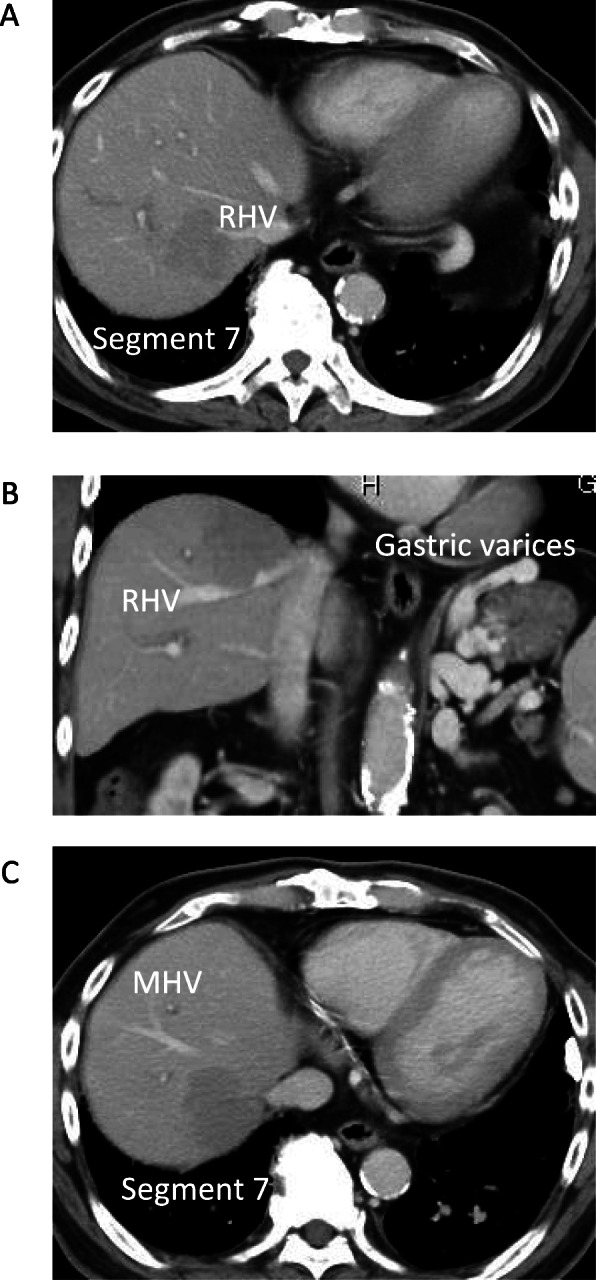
CT imaging of HCC. CT showing the tumor located in segment 7 involving the right hepatic vein, a transverse plane, b coronal plane, and c close to the middle hepatic vein. HCC, hepatocellular carcinoma; CT, computed tomography
